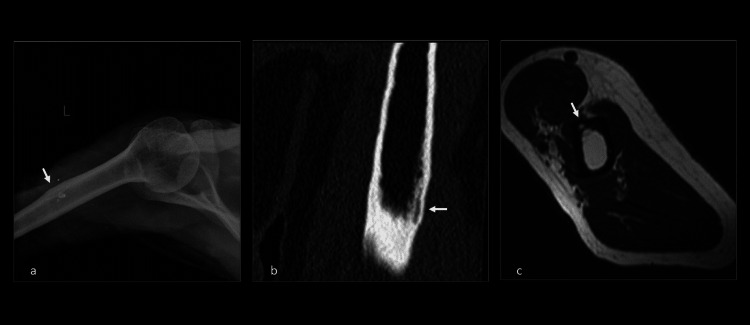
X-ray, CT, and MRI evaluation of the left shoulder. (a) Frontal radiograph of the left shoulder shows an ill-defined area of cortical thickening and sclerosis at the deltoid insertion. (b) Coronal CT of the left humerus shows well-defined cortical thickness, mild irregularity, and intracortical lucencies along the endosteal cortex. (c) Axial T2WI shows small hyperintense foci in the endosteal region along the anteromedial aspect of the humerus at the insertional site of the deltoid. CT, computed tomography; MRI, magnetic resonance imaging; T2WI, T2-weighted image
